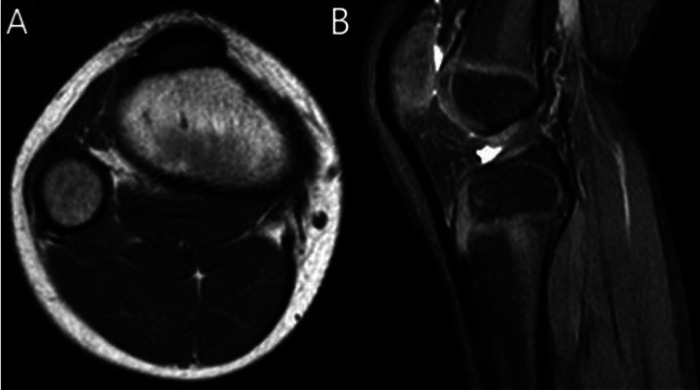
MRI of the right knee. TSE T1w axial (A) and STIR sagittal (B) section of the right side showed normal aspect of the tibial tuberosity.
